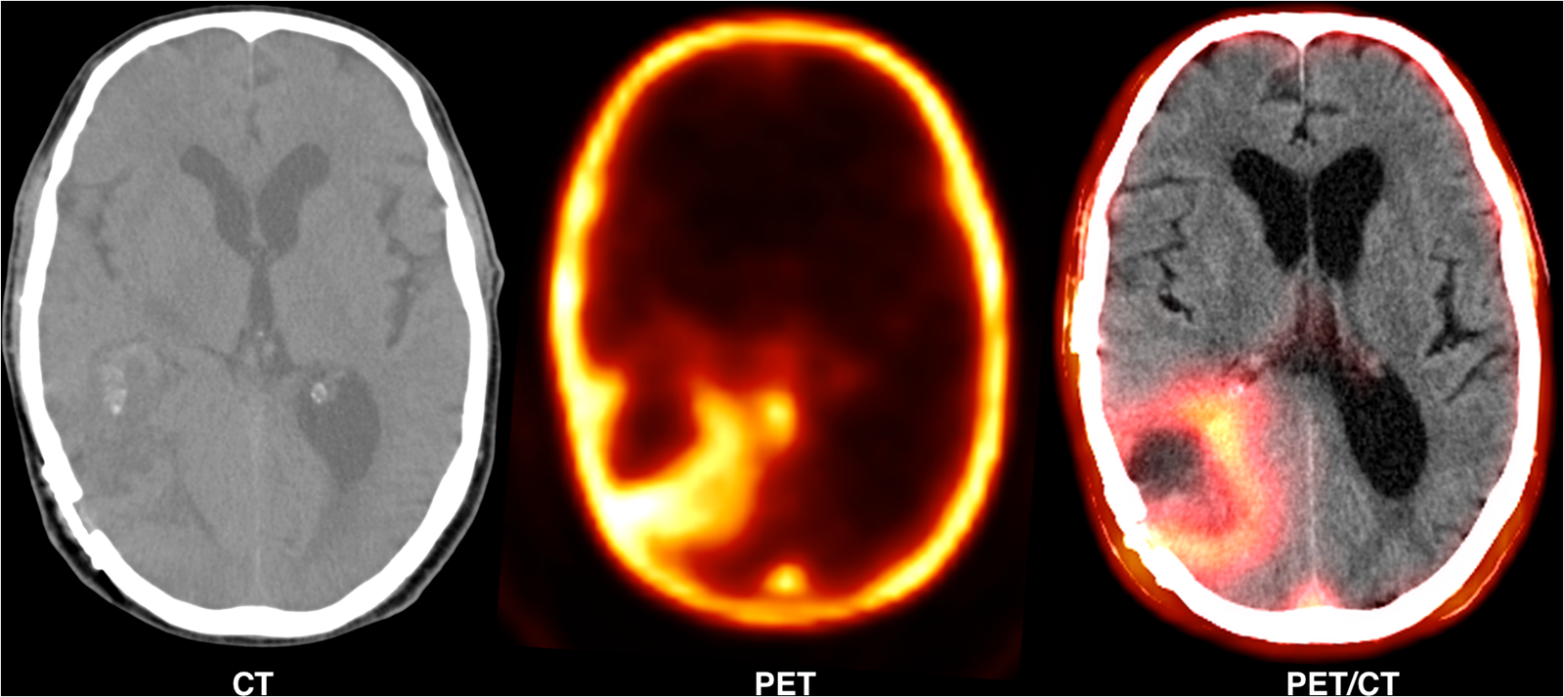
A 62-year-old man with high-grade glioma (WHO IV) that underwent ^68^Ga-pentixafor PET/CT. A large hypo-dense cerebral lesion (7*5.5 cm) was observed in the right parieto-occipital lobe with ^68^Ga-Pentixafor (Pars-Cixafor^TM^) uptake (SUVmax: 1.87), central photopenia (probably necrosis) and good tumor delineation.
